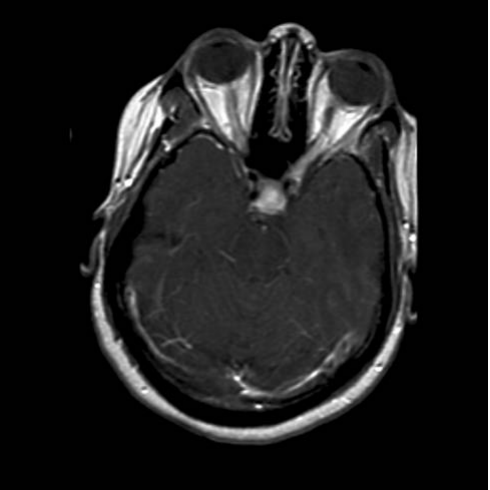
MRI findings one month before initiation of thalidomide, showing marked enhancement of infundibulum (6 mm) and bilateral optic nerves (6 mm).
